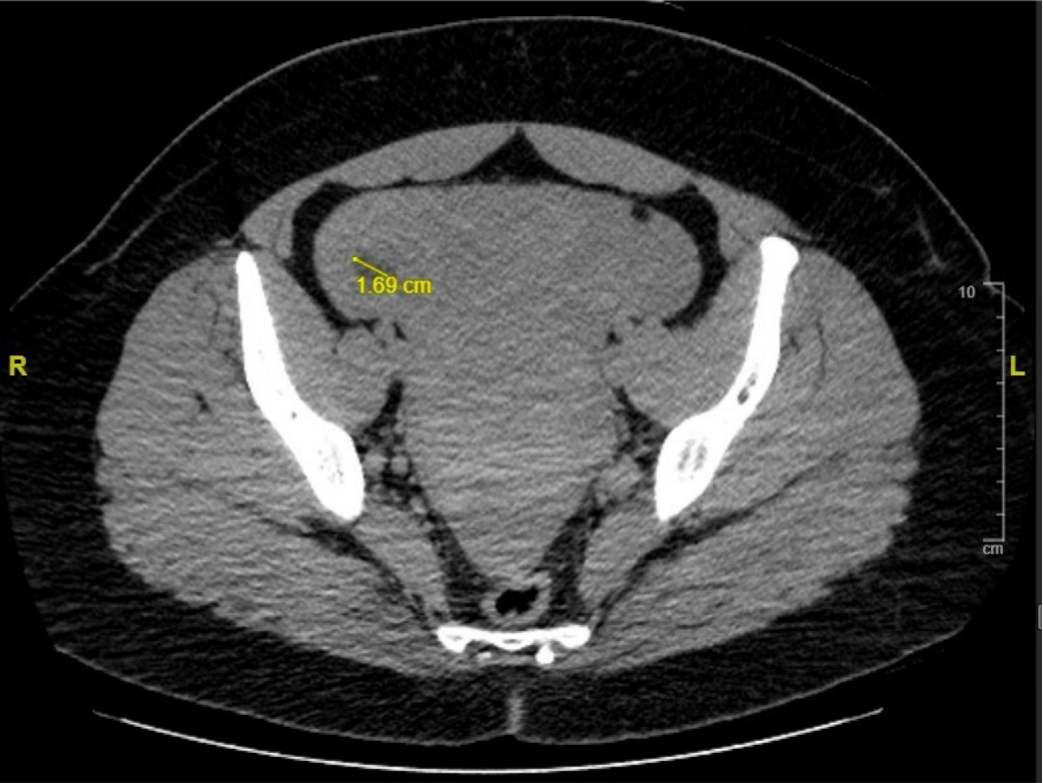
Computed tomography of the pelvis showing a 1.69-centimeter area of low attenuation in the right adnexa. *cm*, centimeter.
